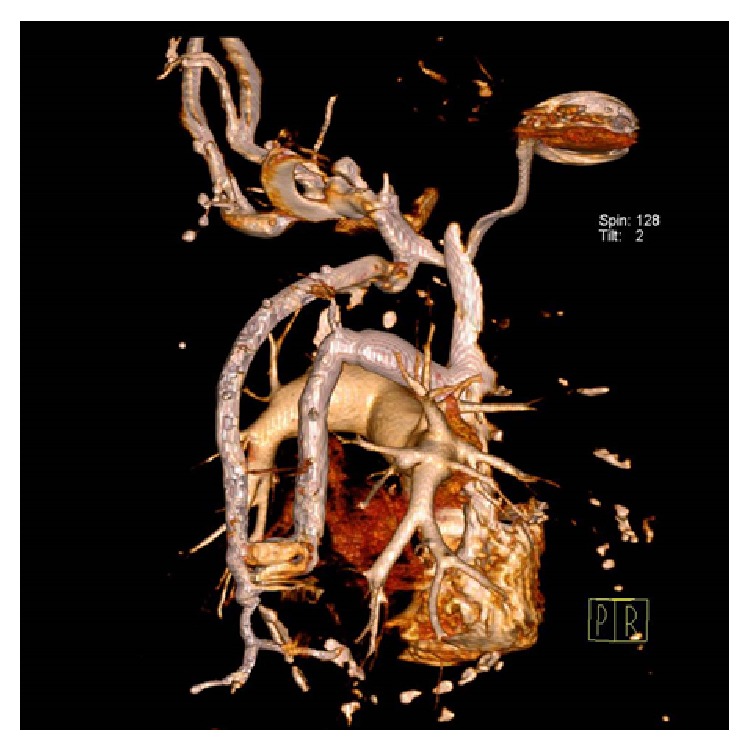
Volume rendering CT image shows the anomaly from the backside.
